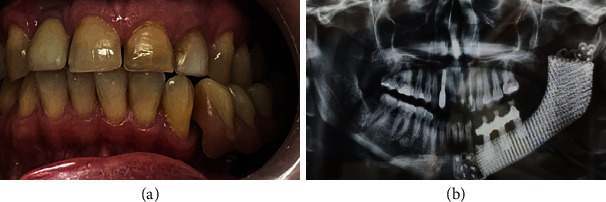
Intraoral view and radiograph after prosthesis.
